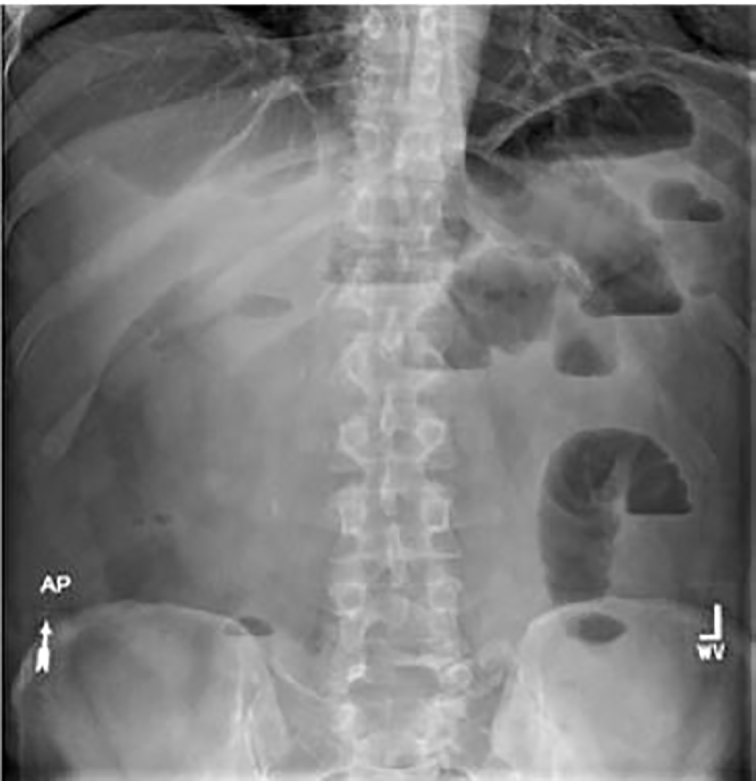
Abdominal radiograph with numerous dilated small bowel loops with air-fluid levels.
